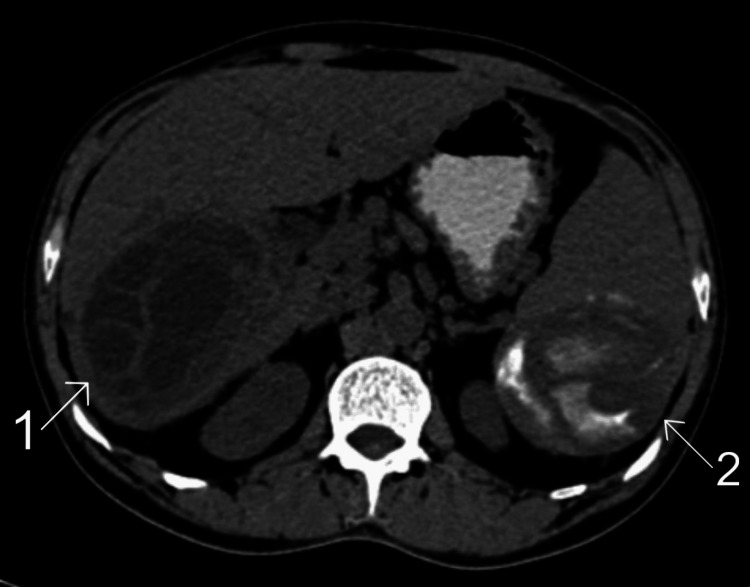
Computed tomography of the abdomen in the patient. 1, hydatid cyst in the liver; 2, hydatid cyst in the spleen
